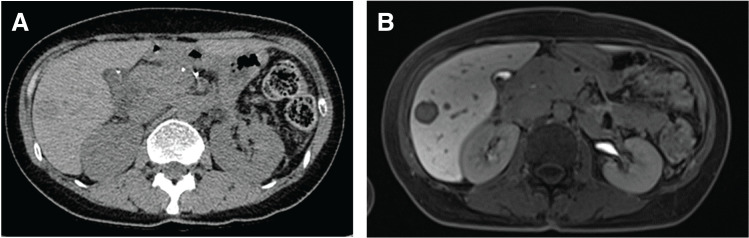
(A) CT image of the S6 segment metastatic liver tumor identified at 7 months after the initial surgery. (B) Gd-EOB-DTPA-enhanced MRI (late phase) showing the same lesion. Gd-EOB-DTPA, gadolinium ethoxybenzyl diethylenetriamine pentaacetic acid-enhanced
